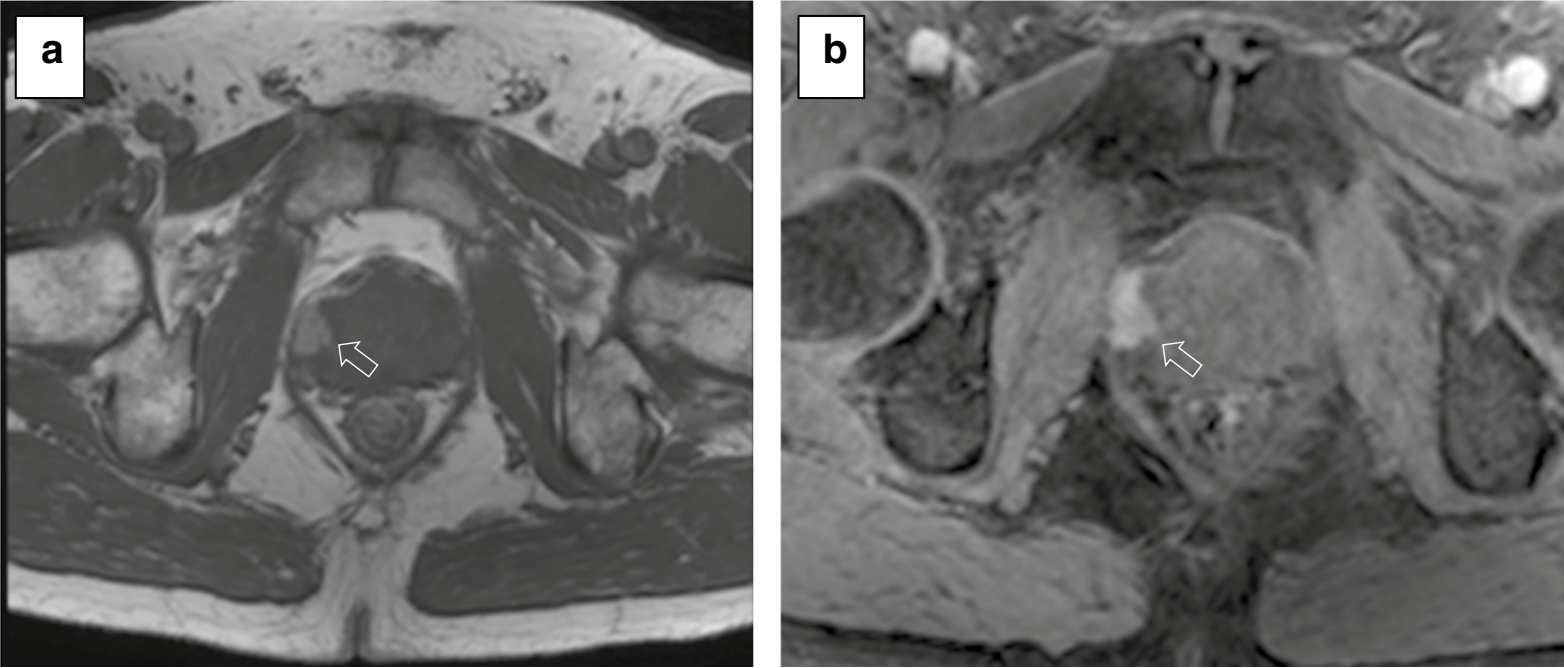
Pre-contrast non-fat suppressed (a) and fat-suppressed (b) T1-weighted images showing a hyperintense focus in the right anterior horn (arrows) in keeping with post-biopsy haemorrhage
